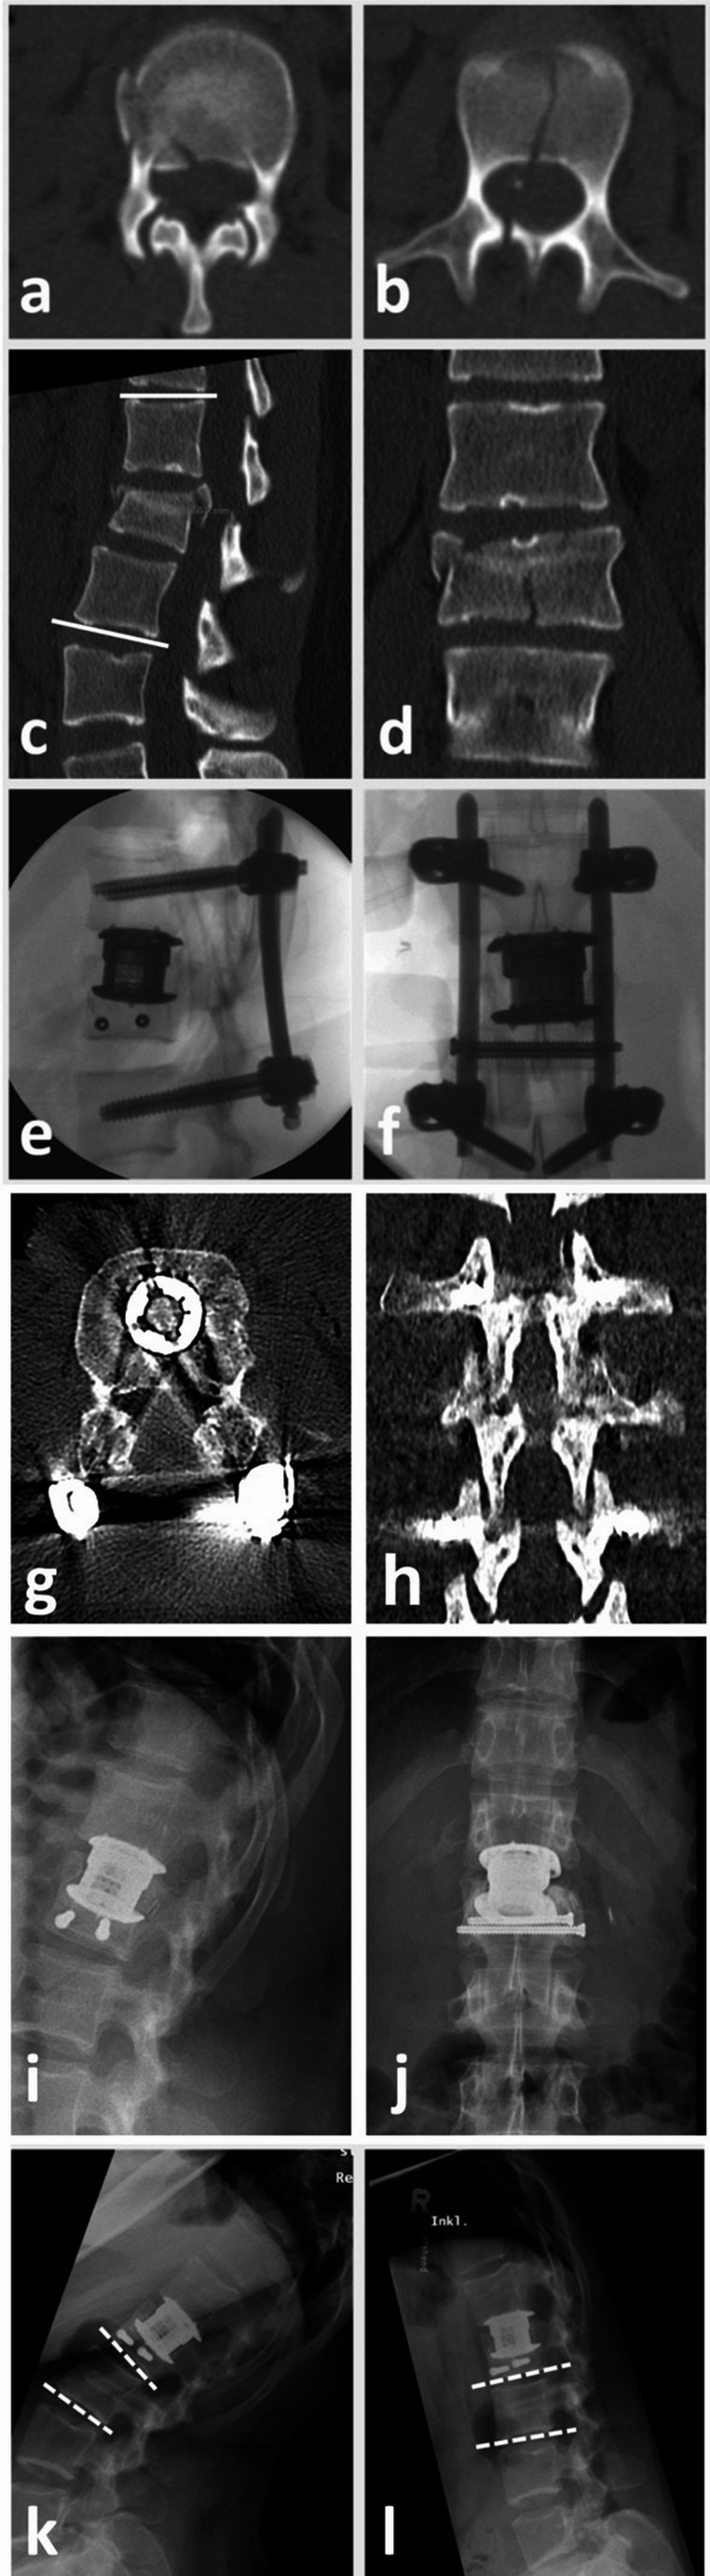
Representative case of a 30-year-old female patient suffering a burst-split fracture of L2 (AO Spine L1-2: B2 (L2: A4; N2; M0)) following a car accident (a-d). Axial reconstruction shows the burst fracture in the cranial region (a) and the split fracture in the caudal region (b) of the vertebral body. The sagittal split size was quantified by measuring the largest gap diameter on coronal CT scans after trauma (d). The patient underwent posterior instrumentation of L1-L3 with monosegmental spondylodesis at the L1/2 level, decompression with clearance of the spinal canal, and sagittal split screw fixation and monosegmental anterior fusion at L1/2 via lumbotomy (e, f). CT imaging after six months showed fracture consolidation of the caudal split, osseous integration of the monosegmental cage, and fusion of the posterior elements (g, h). The patient was scheduled for removal of the posterior instrumentation (i, j). To characterize the inferior intervertebral discs' functionality after removal of the dorsal stabilization, segmental range of motion was assessed comparing the intervertebral disc angle (dashed lines) in flexion (k) and extension (l) lateral standing radiography at 12 months after the injury
